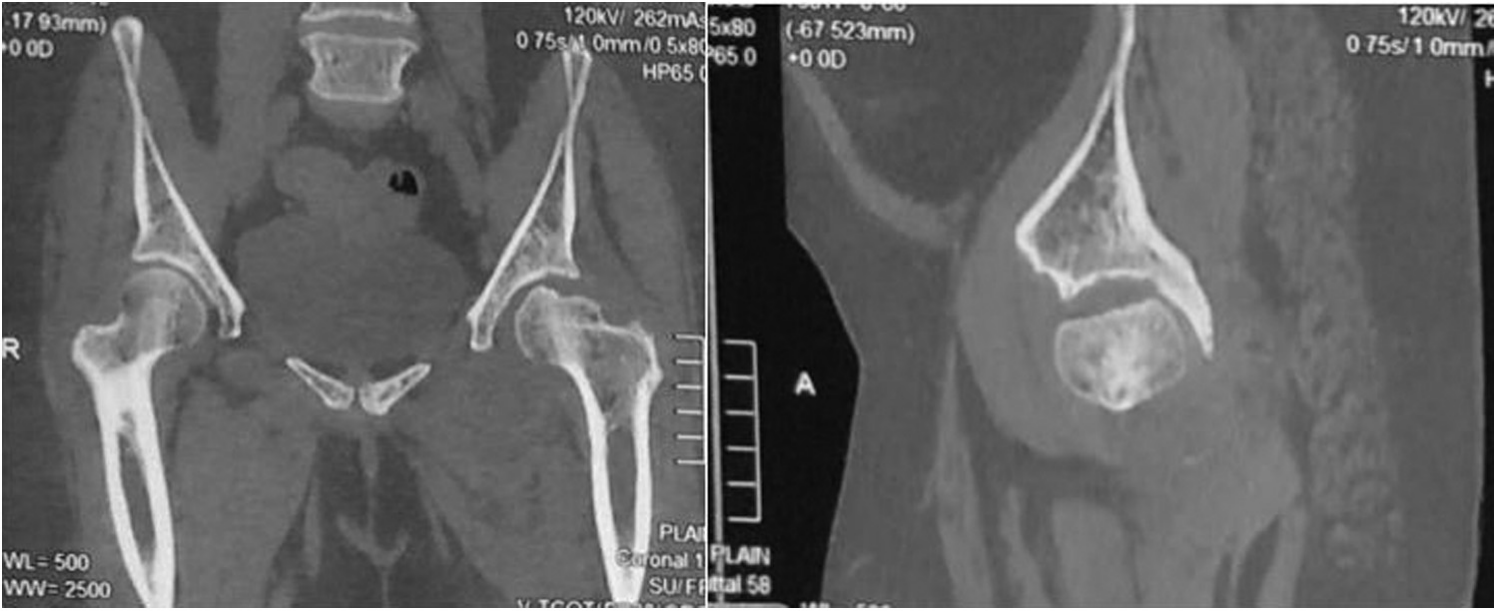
Coronal and sagittal sections of computed tomography scan confirming radiograph findings.
